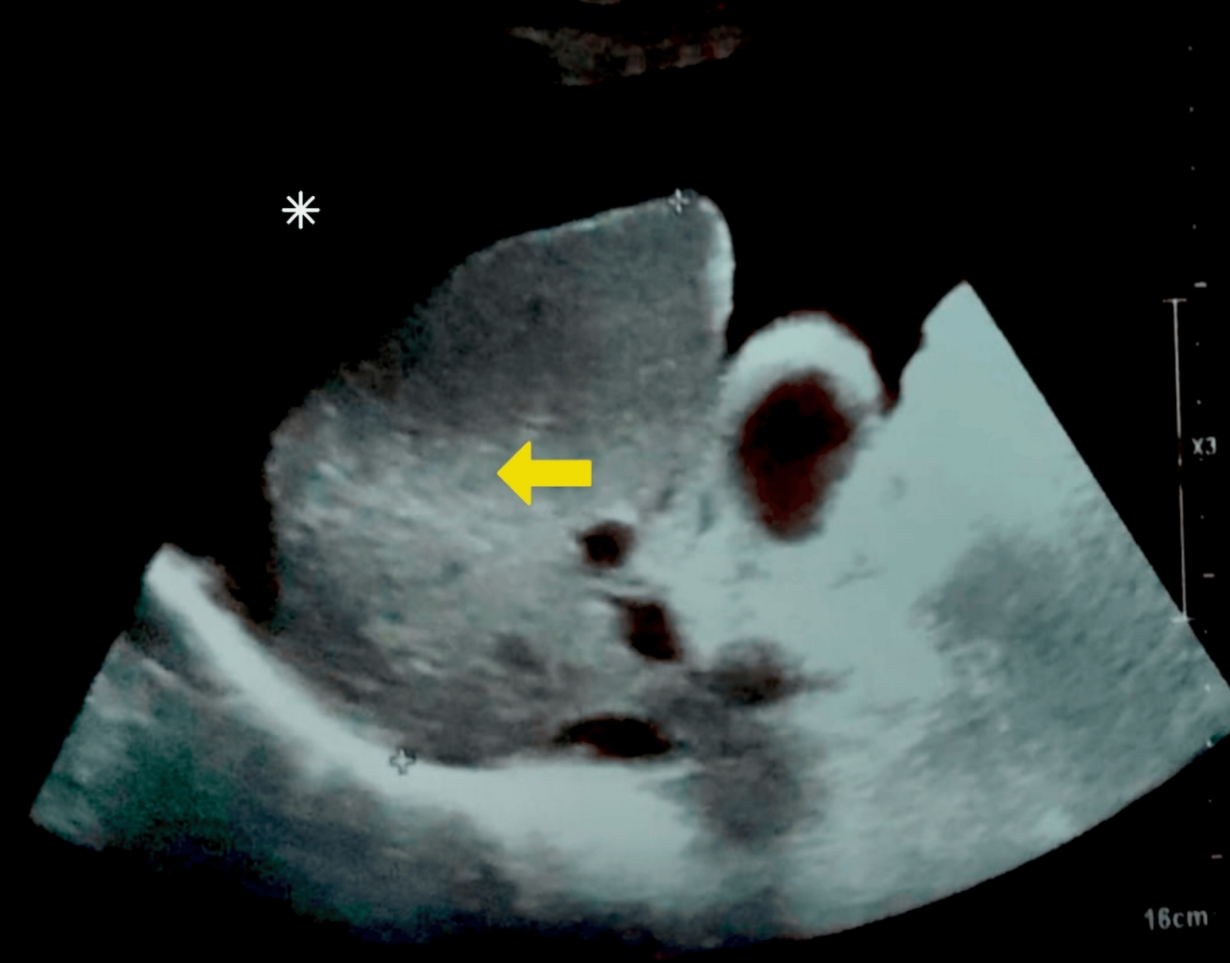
Grayscale ultrasound image of the liver. The yellow arrow indicates the coarse echotexture of the liver, suggestive of underlying cirrhosis. The white asterisk denotes free fluid in the peritoneal cavity, consistent with ascites
